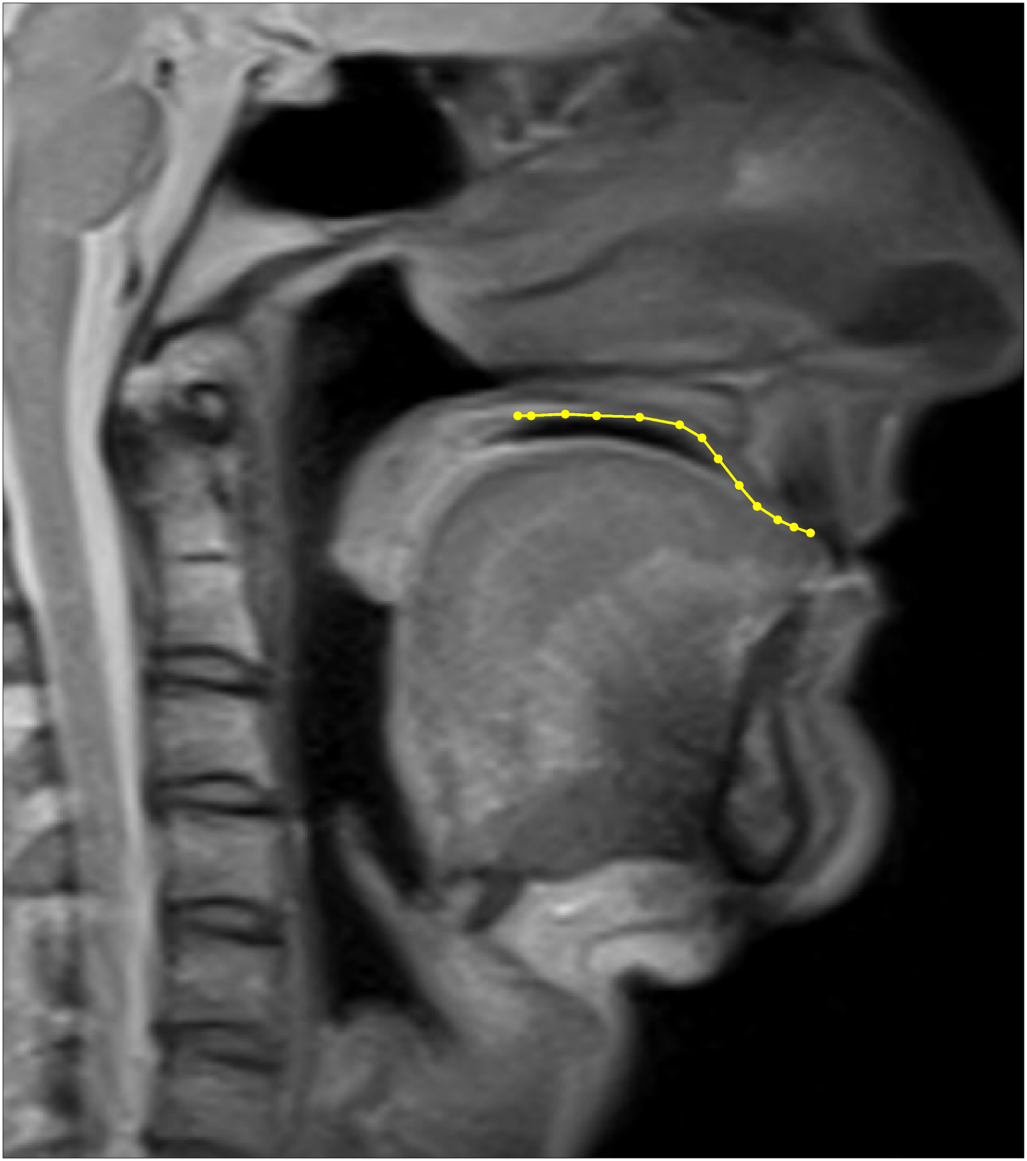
Mid-sagittal MRI scan with tracing. An example of a midsagittal MRI scan (41 years old male) also showing one manual tracing (in yellow; the circles represent the actually sampled points and the lines connect consecutive points).
